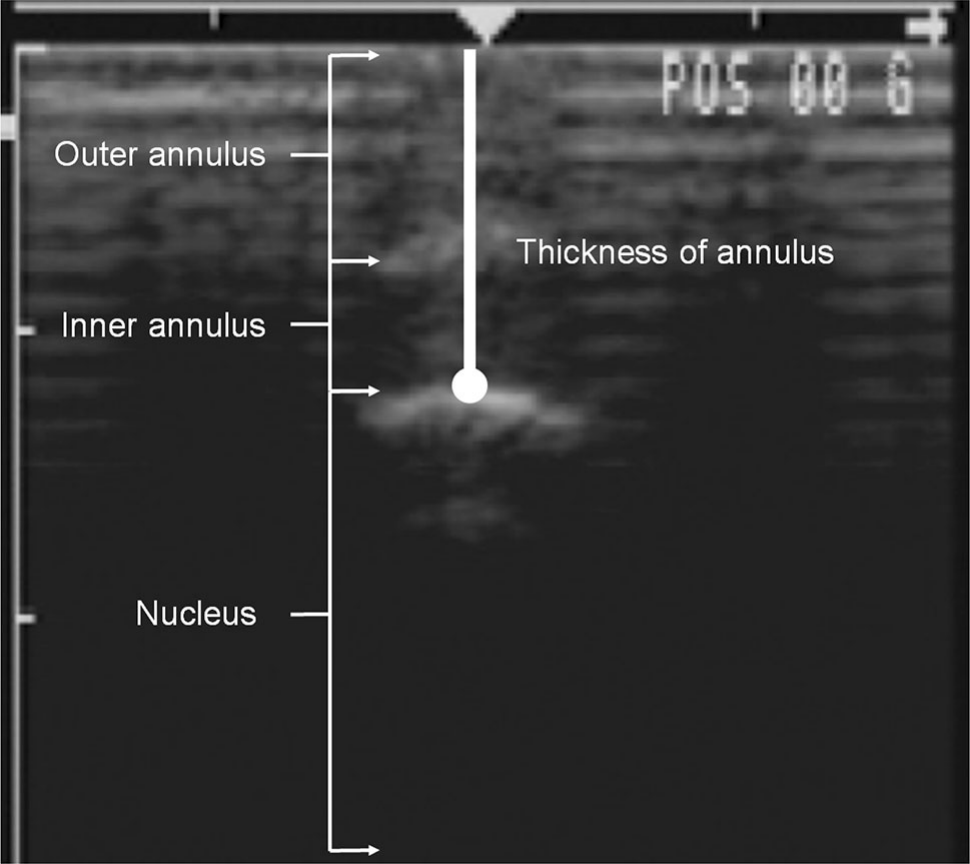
The thickness of annulus fibrosus was measured in sonographic images at different stages of compressive loading. The *left* border of the image is marked in centimeters
